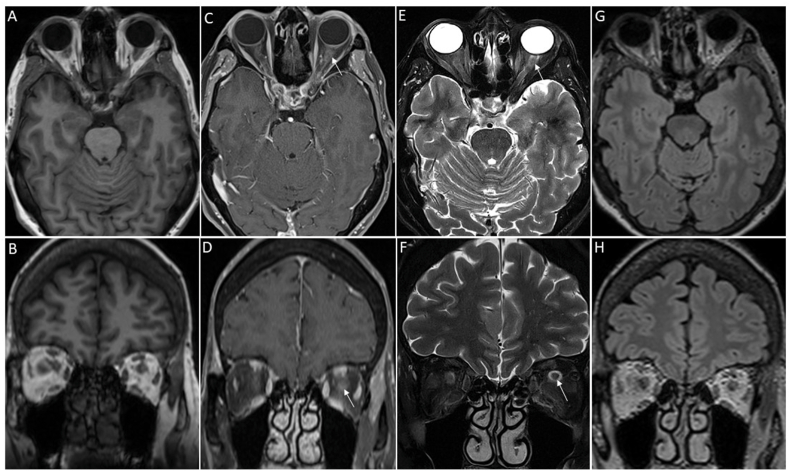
Brain and orbital magnetic resonance imaging (MRI) performed the day of presentation of a 67-year-old woman who had a visual acuity decrease in the right eye associated with bilateral moderate ocular pains occurring with eye movements five days after uncomplicated bilateral cataract surgery. Non-contrast axial (A) and coronal (B) T1-weighted images showing no relevant anomalies. Post-contrast fat-saturated axial (C) and coronal (D) T1-weighted images. There is tortuosity of the right optic nerve. Thickening and contrast enhancement of the optic nerves were observed, predominantly on the left eye (arrows). Axial (E) and coronal (F) fat-saturated T2-weighted images. The left optic nerve shows thickening and hyperintensity (E, arrow). There is dilatation of the subarachnoid spaces of the optic nerve sheaths, predominantly on the left (F, arrow). Axial (G) and coronal (H) T2 Fluid-Attenuated Inversion Recovery (FLAIR) showing no relevant anomalies.
